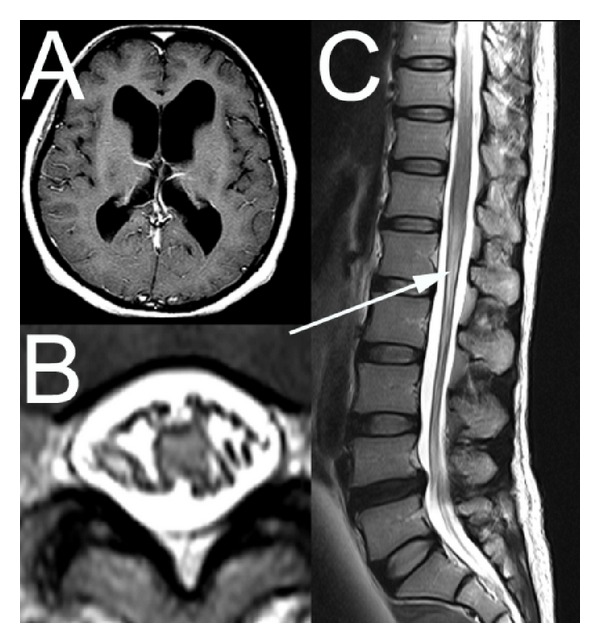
Brain MRI shows marked enlargement of all ventricles (A) and T2-weighted axial (B) and sagittal (C) MR images of the lumbar spine demonstrate increased signal intensity lesion at the level of conus medullaris (arrow). The lesion is diffuse and symmetrical and involves the whole conus medullaris on axial image.
